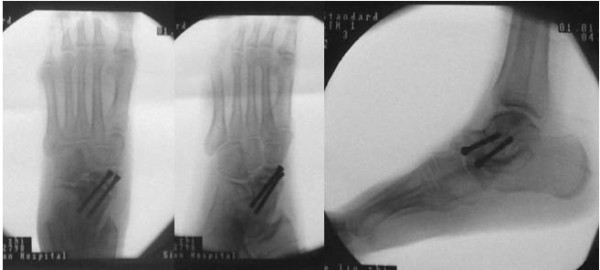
Post-operative X-ray of the talonavicular joint.
